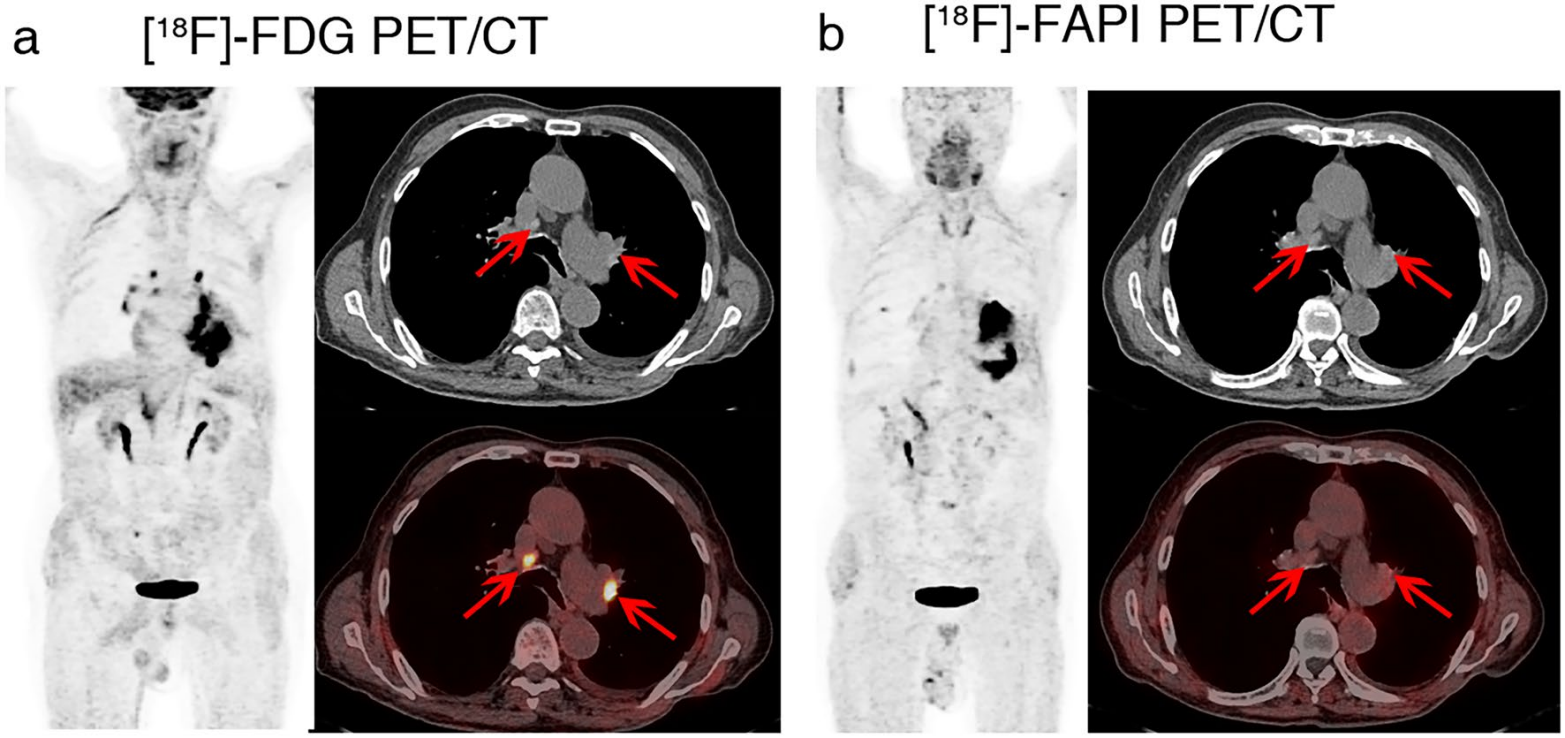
A 79-year-old man with a diagnosis of lung cancer. Lymph nodes in region 4R and 10L with intense ^18^F-FDG uptake (a, red arrows) and no ^18^F-FAPI uptake (b, red arrows) were confirmed as nonmetastatic lymph nodes pathologically
